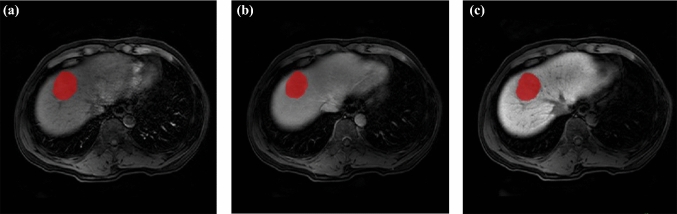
A case of hepatocellular carcinoma (HCC) with Gd-EOB-DTPA-enhanced MRI: a 50-year-old man with pathologically confirmed HCC. The lesion area on each axial slice of arterial phase a, portal vein phase b, and hepatobiliary phase c images was delineated
